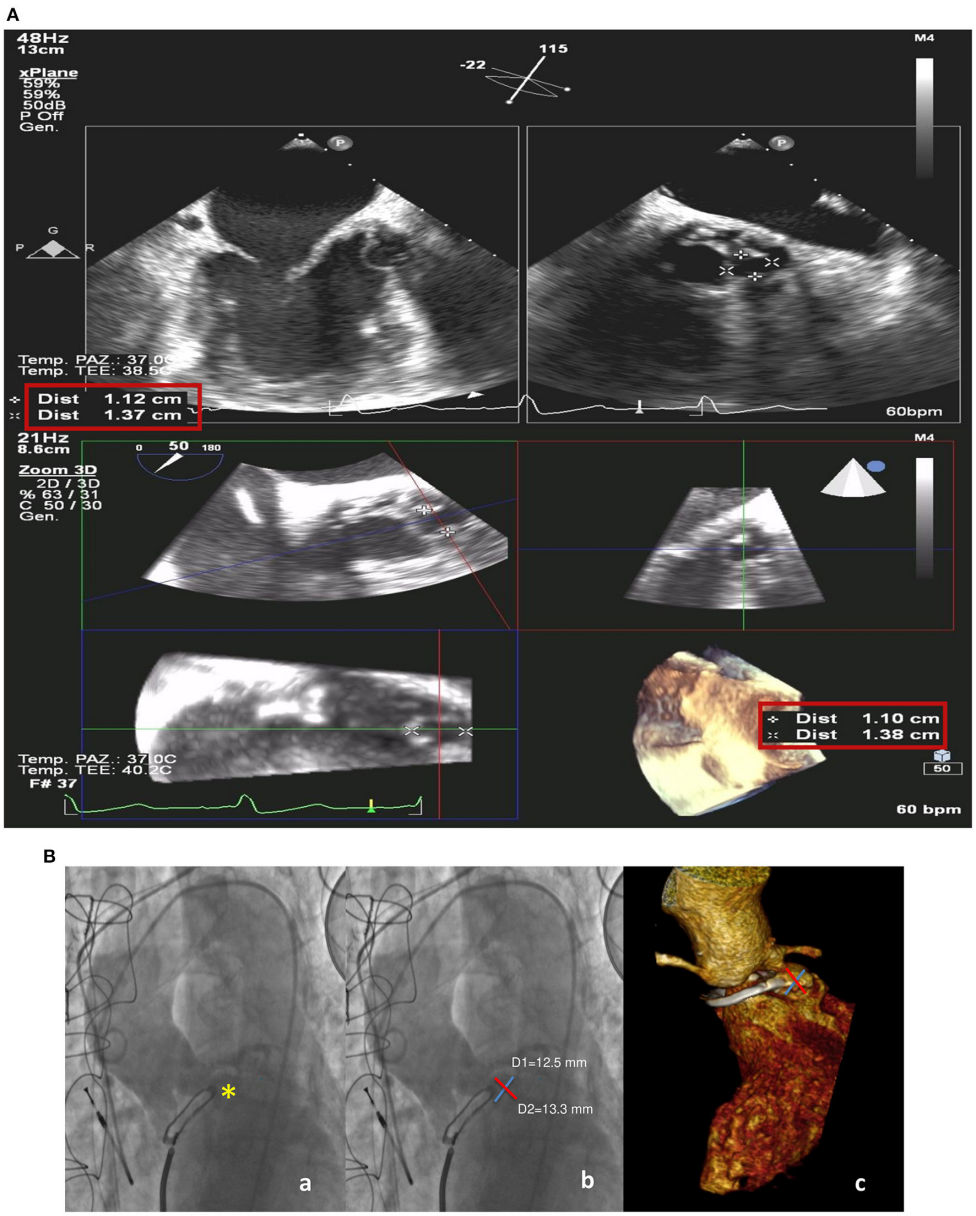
Intraprocedural transesophageal echocardiogram (A). 2D X-plane imaging (upper panels) and 3D (bottom line) with measurements of the paravalvular pseudoaneurysm fistula. Preprocedural ascending aorta angiogram and volume-rendering three-dimensional (3D) multidetector computed tomography angiography (MDCT) (B) showing the paravalvular pseudoaneurysm (yellow star) (a) and its measurements on the two orthogonal axes from angiographic (b) and MDCT images (c).
